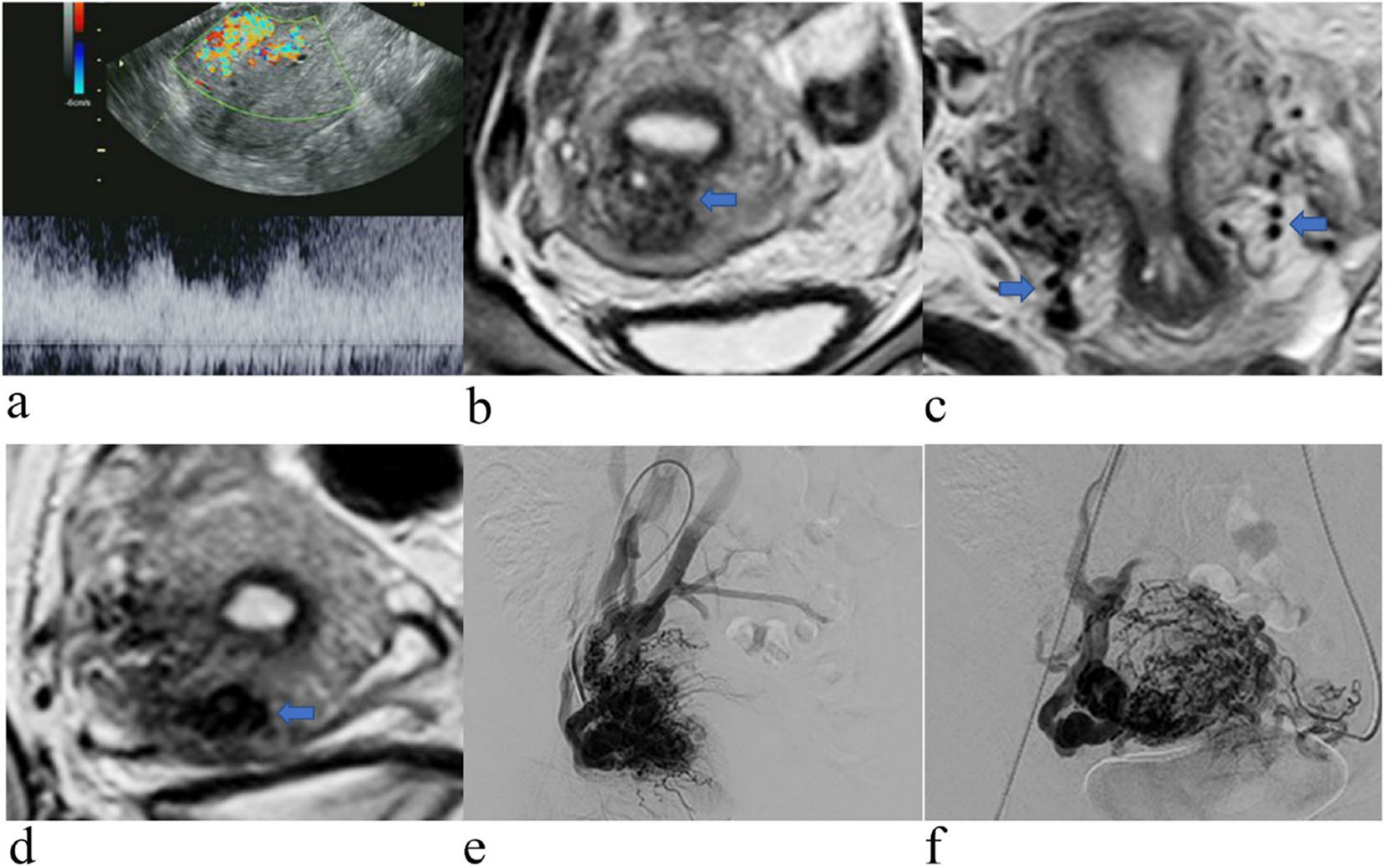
Images obtained of a 29-year-old woman with GTD coexisting with a uterine AVM after chemotherapy. (a) Colour Doppler imaging of the arteriovenous malformation within the myometrium showing brisk, highly expanded flow and pulsed Doppler imaging showing low-resistance, high-velocity flow. (b) Coronal T2-weighted MRI showing a mass located in the myometrium with an unclear boundary and heterogeneous signal (blue arrow). (c) Axial showing multiple vascular-like, irregular, serpentine flow void signs in the parametrium (blue arrow). (d) Coronal T2-weighted MRI showing multiple vascular-like, irregular flow void signs in the myometrium (blue arrow). (e,f) Pelvic angiogram of the left and right uterine arteries revealed simultaneous filling of the right draining veins in the early arterial phase, which is a definitive diagnostic finding of uterine AVMs.
